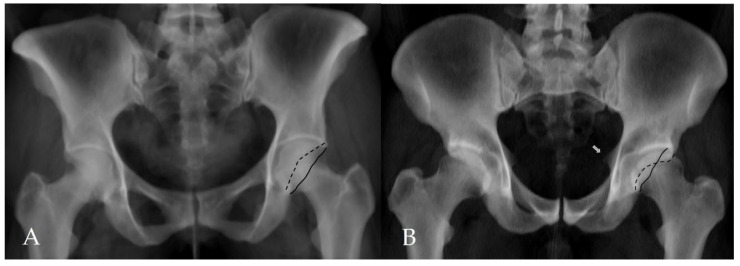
Assessment of additional morphological parameters of femoroacetabular impingement on a CT-based virtual pelvic radiograph. (A) Negative acetabular crossover sign. The black solid line represents the posterior acetabular wall, and the black dashed line represents the anterior acetabular wall. (B) Positive acetabular crossover sign with an associated ischial spine sign (white arrow), suggestive of pincer-type femoroacetabular impingement and acetabular retroversion.
